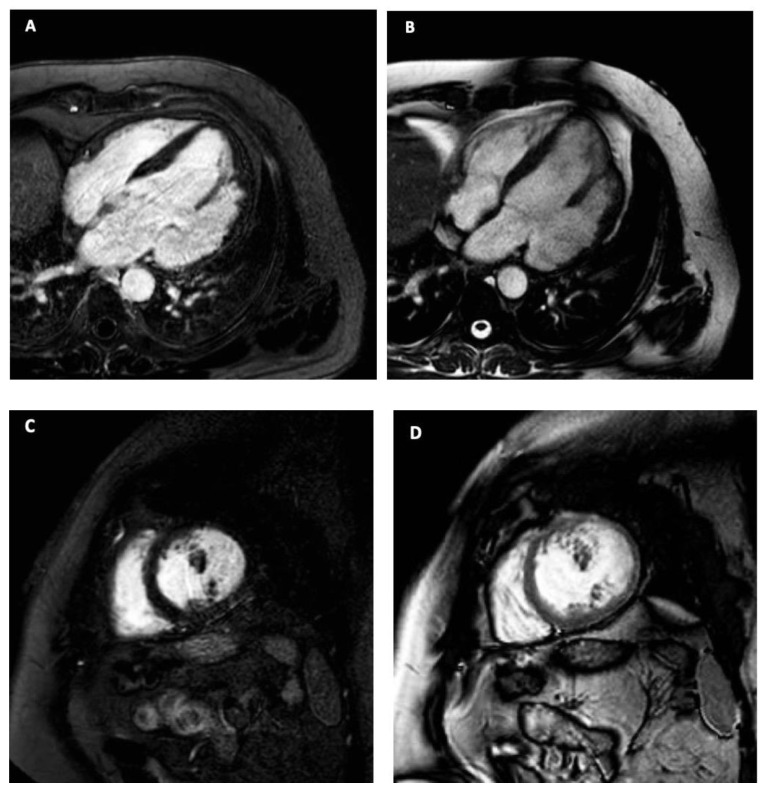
MRI findings. Long-axis 4-chamber LGE (A) and Cine Steady-state free-precession (SSFP) (B). Additionally, short-axis (C) LGE and (D) SSFP images show an apical accessory cavity connected to the ventricle in the absence of a thrombus or communication with the right ventricle.
